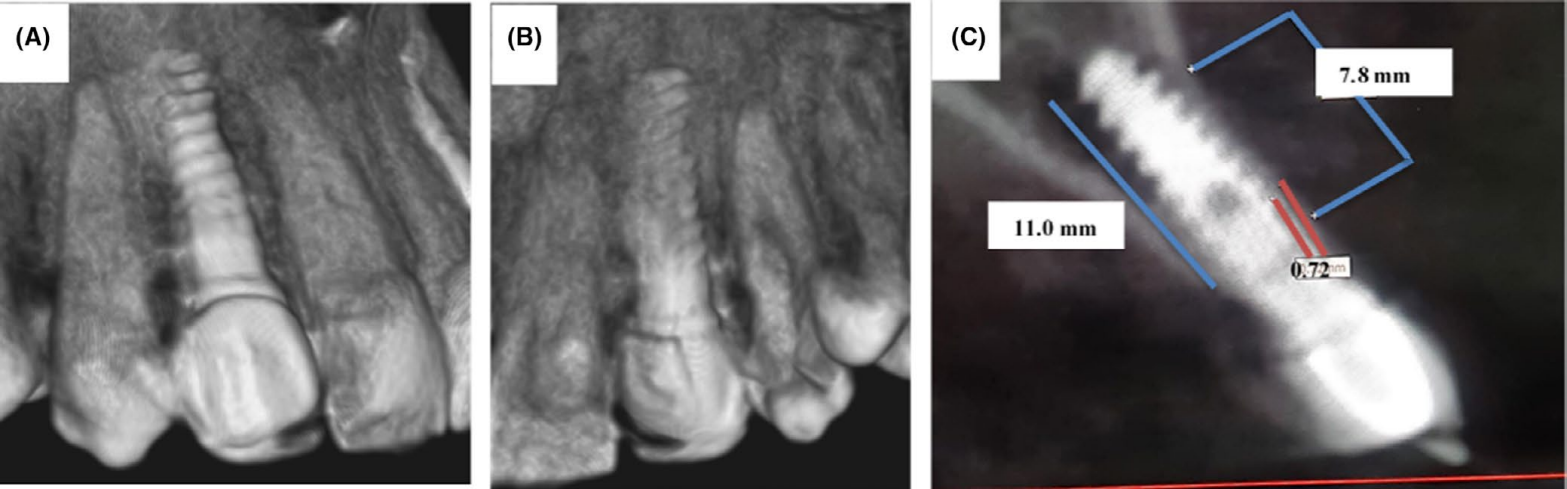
3D composition of the implant site after treatment in labial view (A) and palatal view (B). (C) The CBCT imaging of impant site showing the measurements of bone height in labioplatal view after treatment
